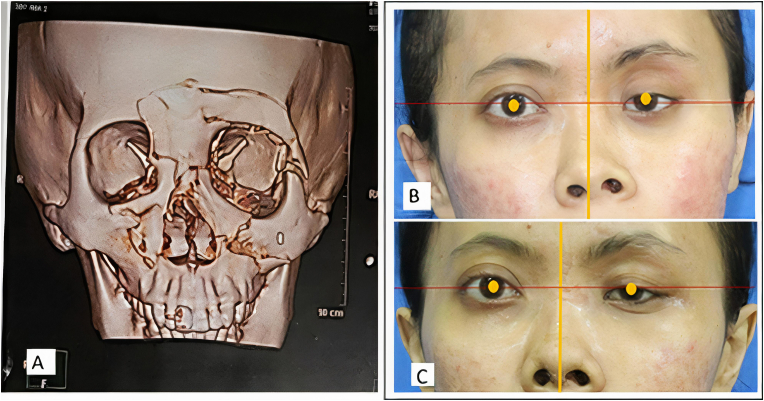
(A) Pre-surgery 3D facial CT-Scan; (B) Assessment of vertical dystopia by connecting the horizontal line between the two pupils and assessing the position of the pupils is not parallel in the horizontal plane. Preoperative facial radiograph showing the position of the pupil in a horizontal plane not parallel. (C) Postoperative facial photograph showing the position of the pupil in a more parallel horizontal plane.
